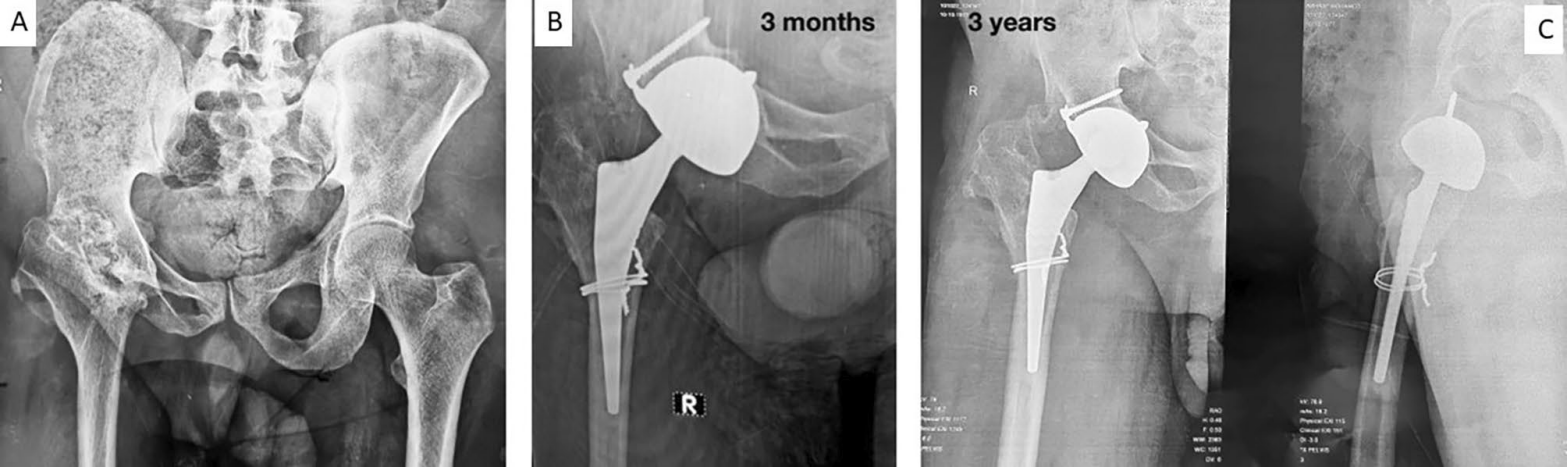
53 years old male patients who had hip arthritis secondary to septic arthritis in childhood and pelvic support osteotomy (A) preoperative x-ray with advanced arthritis limb length discrepancy and valgus position of the proximal femur (B) Libra cementless stem and Novae® E TH DM cup, intraoperative crack of the proximal femur during stem insertion was observed and protected with wires (C) 3 years post-operative with full bone ingrowth around the stem
